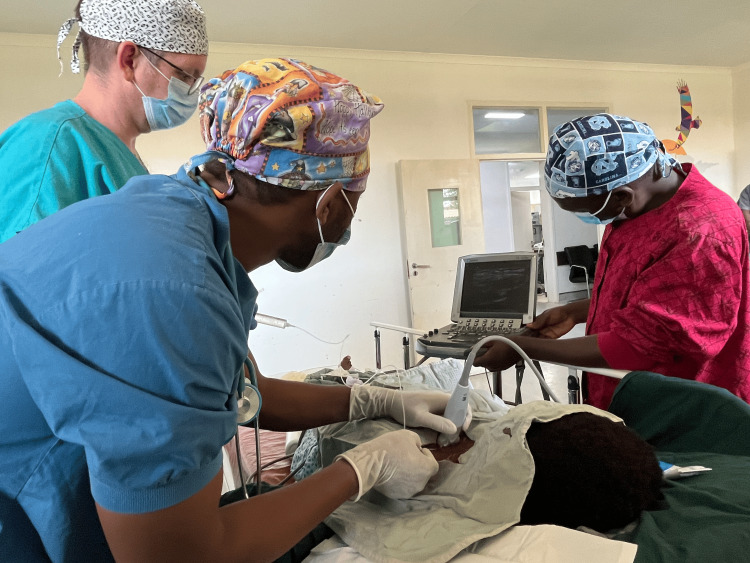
Hands-on education of POCUS being utilized to place a peripheral nerve block Consent was obtained from all healthcare workers shown POCUS, point-of-care ultrasound
